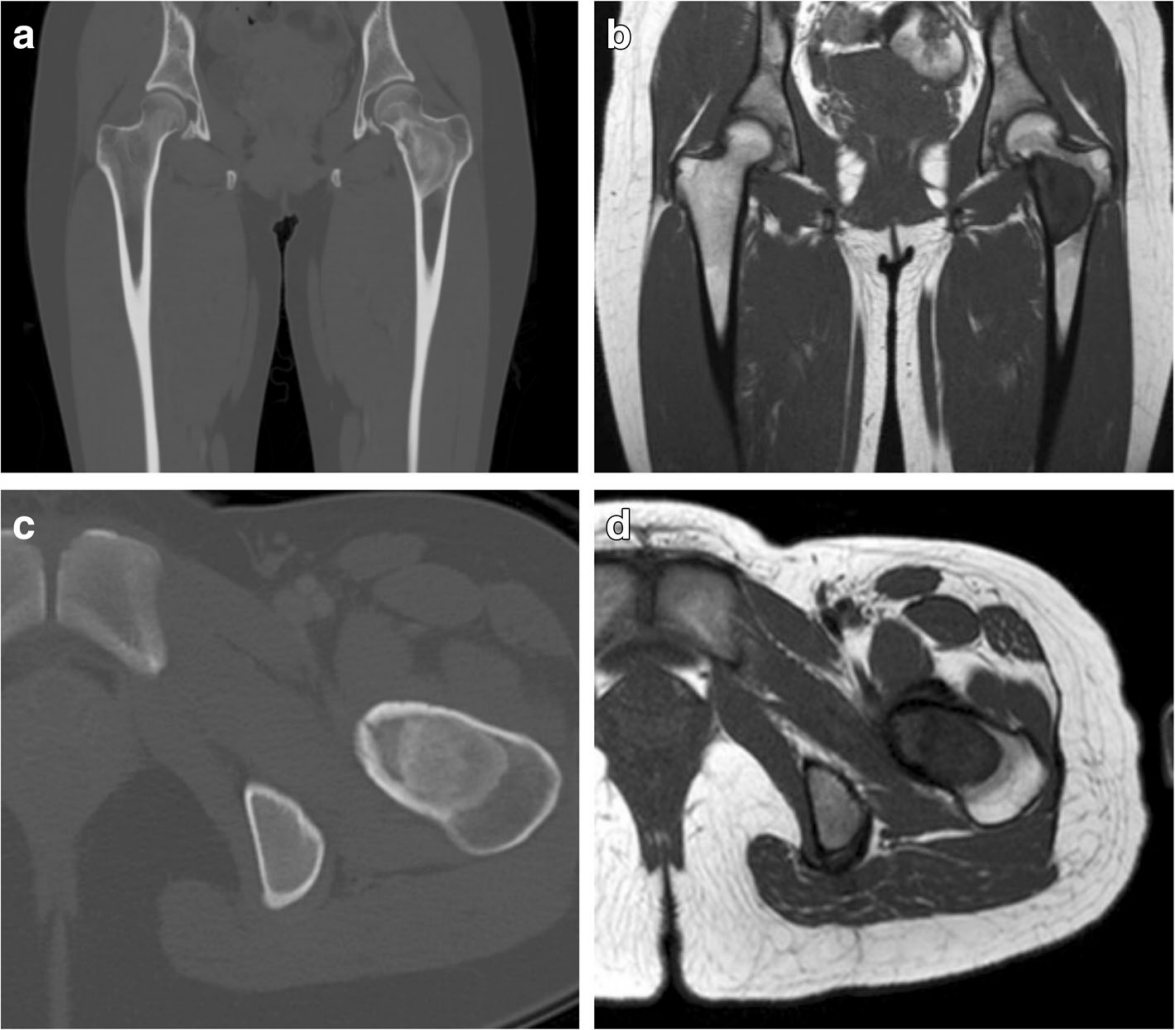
Preoperative CT and magnetic resonance images of proximal femoral lesion. a and b CT, coronal and axial views of proximal femur. c and d T1-weighted magnetic resonance images, coronal and axial views of proximal femur
